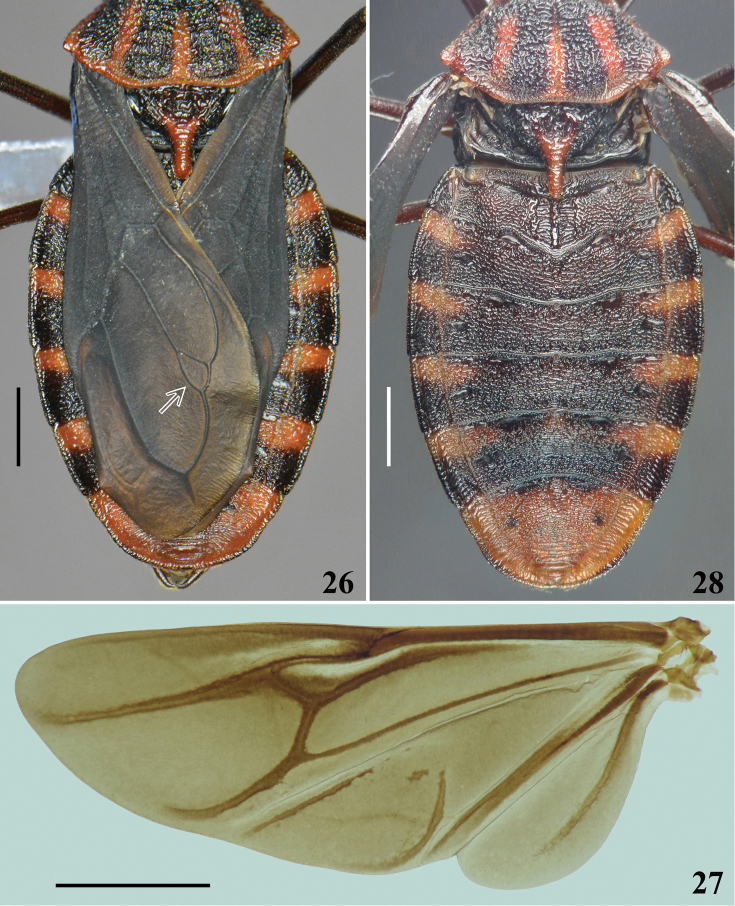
Volesusnigripennis*, male, dorsal view 26 distal portion of pronotum, scutellum, hemelytra and connexivum, arrow points to a small additional cell at approximately apical fourth of the cubital vein 27 left hind wing 28 distal portion of thorax and abdomen (wings moved away). Scale bar: 2.0 mm.
